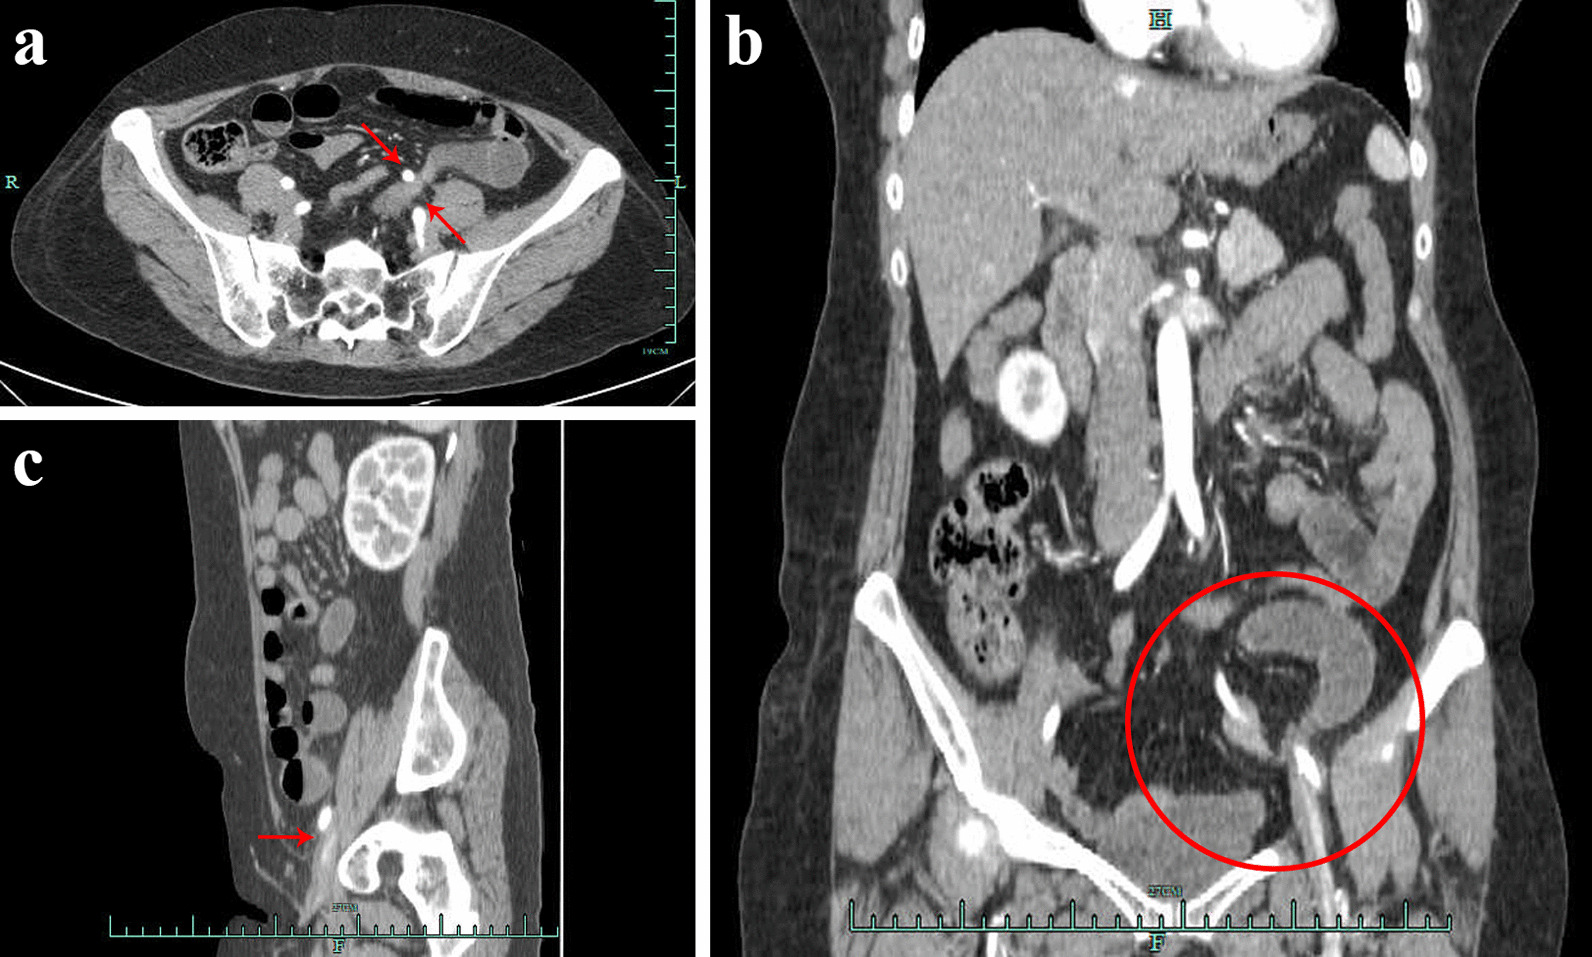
The preoperative contrast-enhanced CT scan. a axial view showing herniation of small bowel with abrupt reduction of caliber (red arrows) between left EIA and psoas major. b coronal view showing the site of herniation and distension of proximal bowel loop (red circle). c sagittal view showing herniation of small bowel (red arrow) beneath the left EIA
